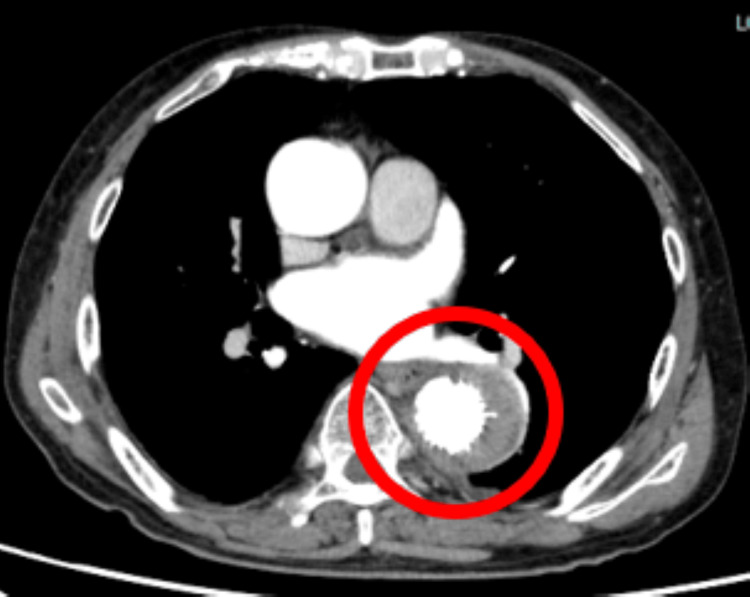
The horizontal slice of chest CT CT image after vascular stenting on 33 days after admission (red round). No signs of infection or endoleak are observed.
